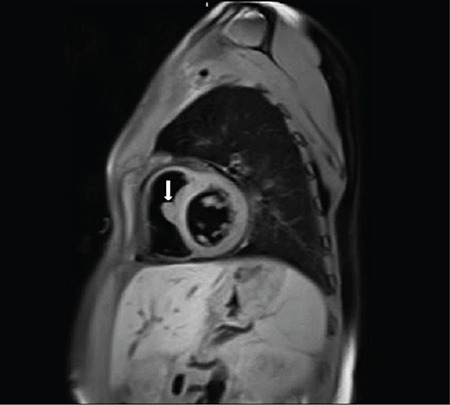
Histophatologic features of the biposy material. Sagittal section with T2 sequences of cardiac magnetic resonance imaging scan indicates a 10x15 mm mass in the cardiac interventricular septum (white arrow).
